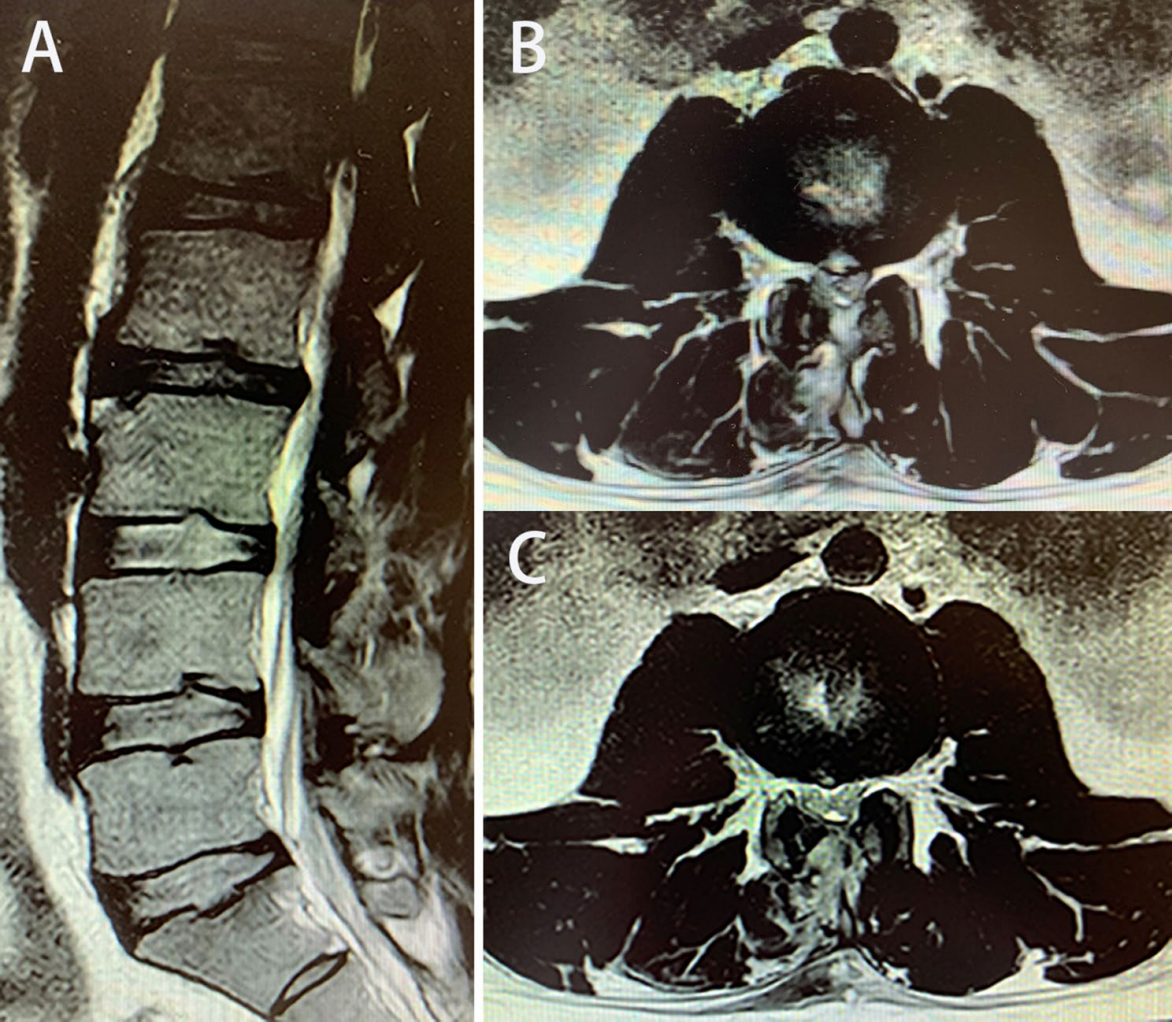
Postsurgical MRI showing that the herniated L1-L2 and L2-L3 intervertebral discs were removed completely and the dural sac was restored to swell. (A) Sagittal view. (B and C) Axial views of L1-L2 and L2-L3.
